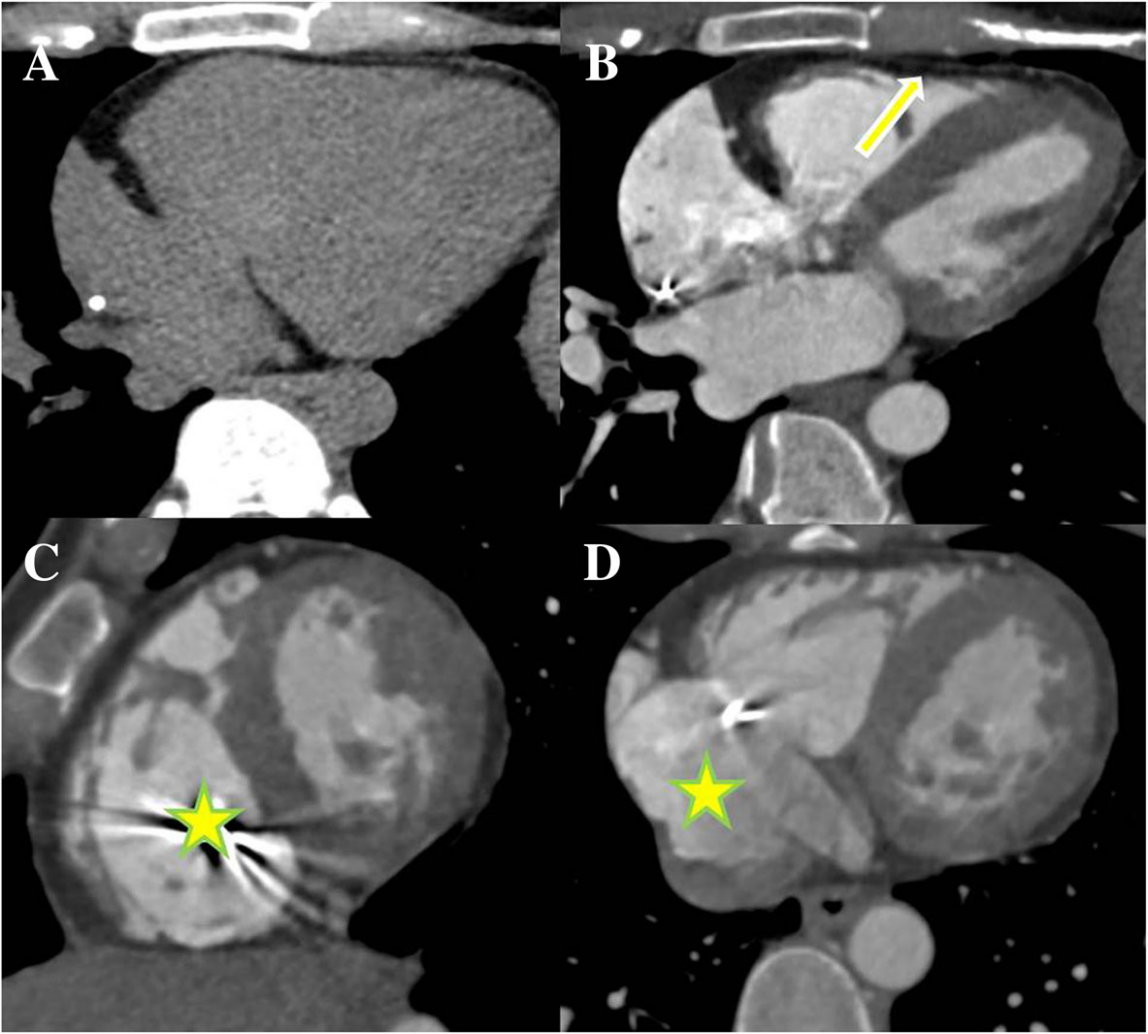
Cardiac CT images of III:1 patient. Four-chamber view of non-enhanced image (a) and enhanced image (b). Thinning of the anterior wall of the right ventricle with extensive fatty infiltration (arrow) could be noted. The short-axis view (c) demonstrated dilation of the right ventricle (star). Enlargement of the right atrium (stars) was shown on the axial view (d)
